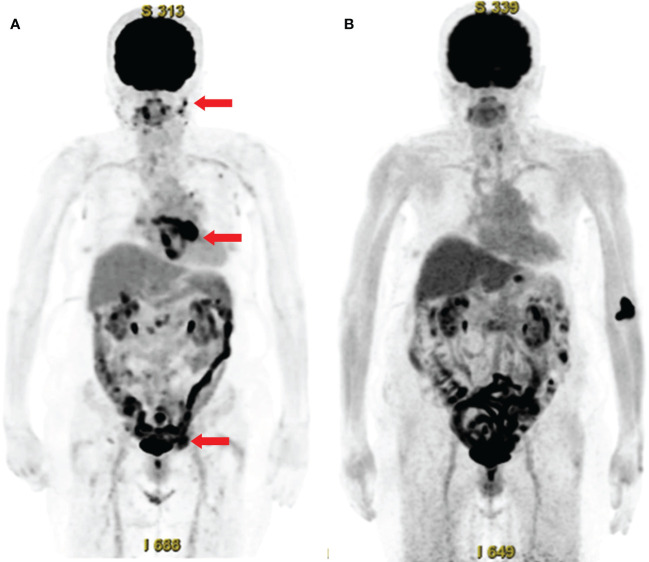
Whole-body ^18^F-fluorodeoxyglucose (FDG)-positron emission tomography with computed tomography (PET-CT) before and after treatment. (A) FDG-PET-CT before treatment, showing abnormal FDG uptake in the facial skin, peri-atrial, pelvic (arrows), cervical, axillary, and inguinal lymph nodes, and colon. (B) Follow-up FDG-PET-CT after treatment, showing the disappearance of abnormal FDG uptake.
